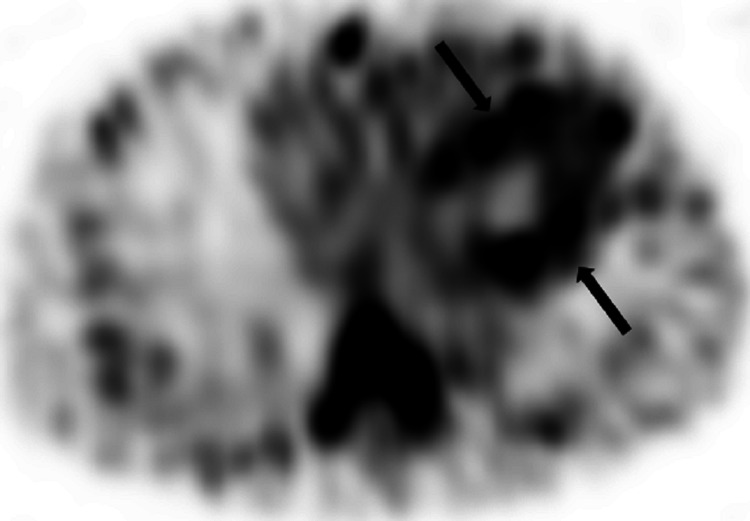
Technetium-99m pyrophosphate scintigraphy- SPECT SPECT of the chest shows uptake in the myocardium (arrows).
